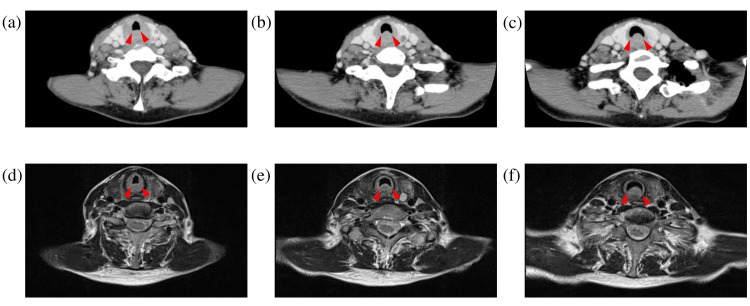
CT and MRI images (a–c) Contrast-enhanced CT scans of the tumor; (d–f) T2-weighted MRI scans of the tumor. Red arrows indicate the bulging posterior tracheal wall and the iso-dense circumferential lesion extending from the subglottis to the upper trachea.
